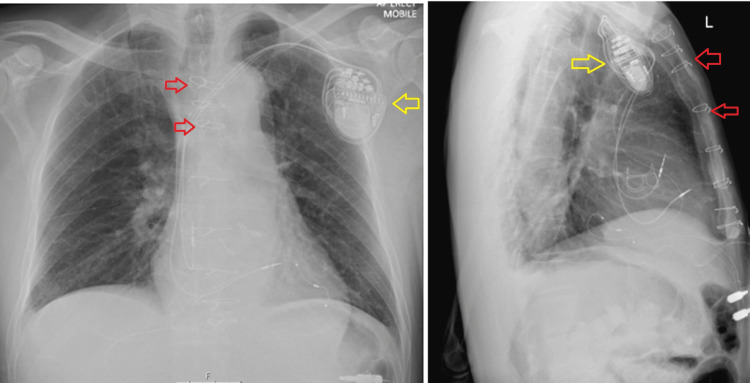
Postoperative X-ray showed thoracotomy suture (red arrows) performed to repair the aortic dissection with the permanent pacemaker inserted (yellow arrows).
